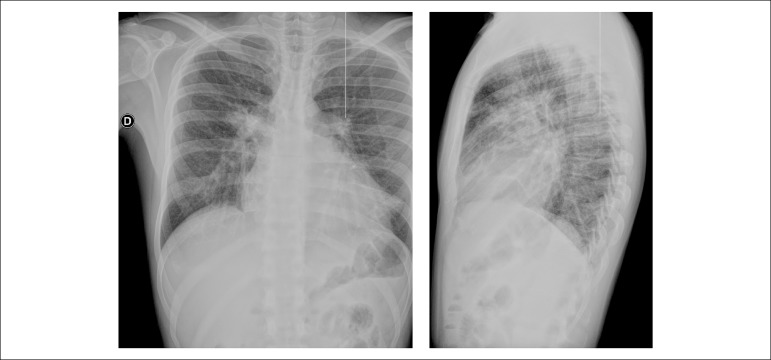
X-rays: global cardiomegaly, larger increase of the right ventricle.
